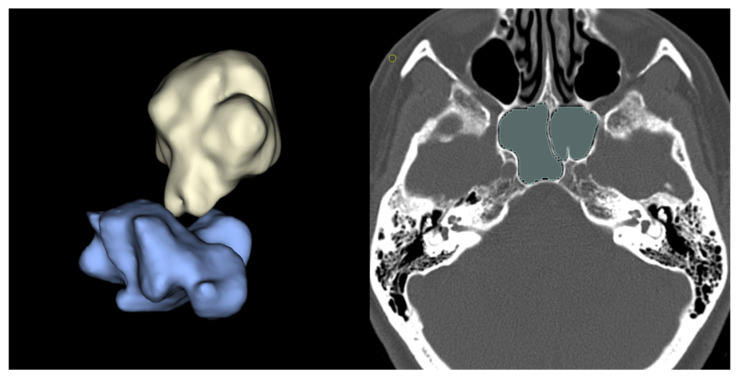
On the (left), a 3D representation of the sphenoidal sinus (light blue) and tumor (yellow). On the (right), the segmentation (green) of the sphenoidal sinus on CT scan (bone window).
